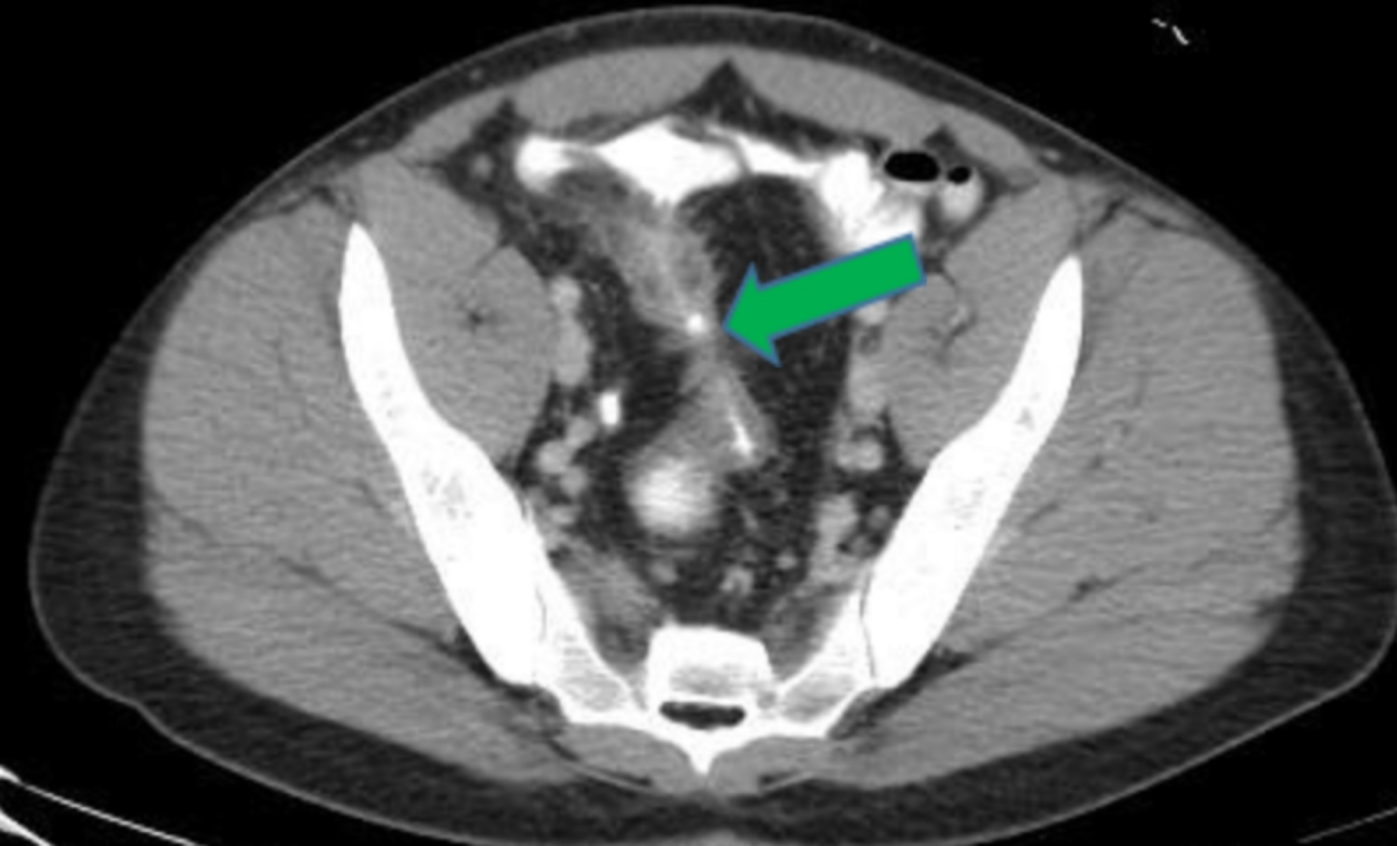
CT scan showing fistula connecting bowels indicated by the green arrow
